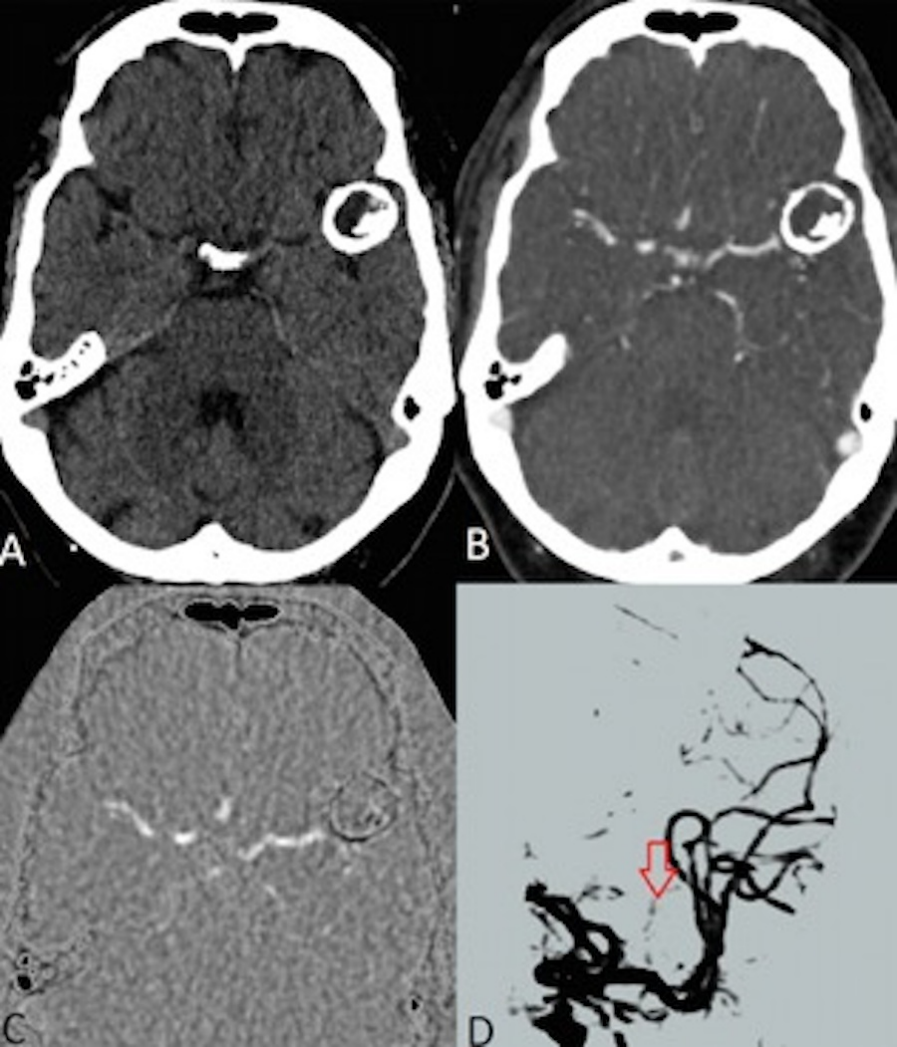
Images from the 4D-digital subtraction CT angiogram. The non-contrast head CT (A) shows a partially calcified aneurysm with a non-calcified component along the anterior/medial wall. An image from the late arterial/early venous phase of the contrast enhanced portion of the study (B) shows no evidence of contrast opacification within the non-calcified component. The two studies are digitally subtracted (C) to further demonstrate the lack of contrast opacification. The data from the arterial, parenchymal, and venous phases are compiled to make a digitally subtracted three dimensional, temporally resolved CT angiogram that looks similar to the conventional angiographic images (D). No contrast fills the aneurysm lumen. The calcified, incompletely subtracted wall (red arrow) is all that can be seen of the aneurysm.
